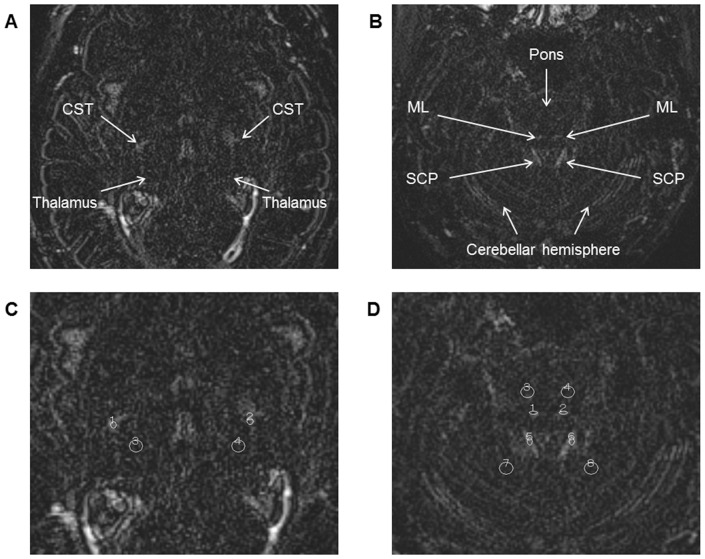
The regions of interest setting on plain-heavily T2-weighted three-dimensional fluid-attenuated inversion recovery (P-hT2w-3D-FLAIR) images. Axial, plain, heavily T2-weighted three-dimensional fluid-attenuated inversion recovery (hT2w-3D-FLAIR) images obtained from a single patient without gadolinium injection. Images on the left side (A and C) are at the level of the thalamus (Th) and images on the right side (B and D) are at the level of the trigeminal nerve. The corticospinal tract (CST), medial lemniscus (ML), and superior cerebellar peduncle (SCP) are visualized as areas of high signal intensity (A and B). Images C and D are trimmed magnifications of images A and B, with regions of interest (ROI) setting. Oval 5-mm^2^ ROI were set on the CST, ML, SCP, and circular 15-mm^2^ ROI were set on the Th, pontine parenchyma (PP), and cerebellar parenchyma (CP) (C and D). To evaluate the signal contrast between white matter (WM)-tracts and contiguous brain parenchyma, the following signal intensity ratios between WM-tracts and contiguous brain parenchyma were calculated: the ratio of the signal intensity of the CST to the signal intensity of the Th on the same side (CThR), the ratio of the signal intensity of the ML to the signal intensity of the PP on the same side (MPPR), and the ratio of the signal intensity of the SCP to the signal intensity of the CP on the same side (SCPR).
